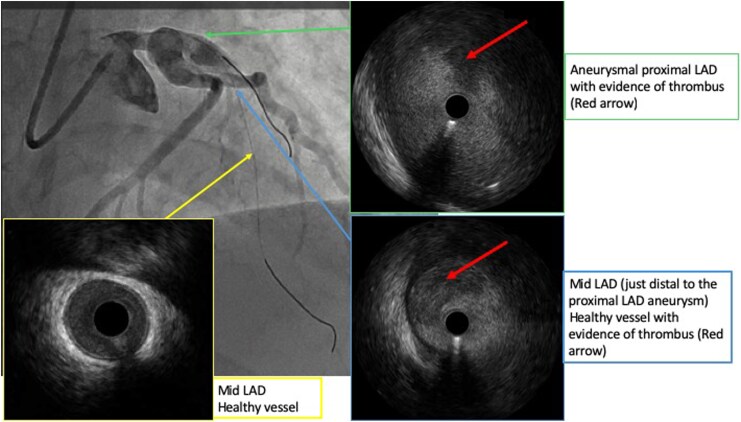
Intravascular ultrasound (IVUS) stills demonstrating a true aneurysm with thrombus without atherosclerosis.
